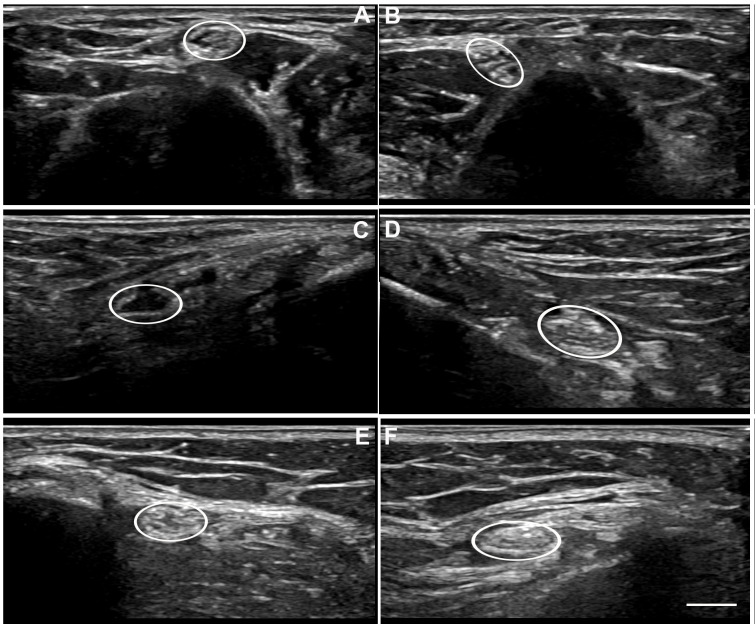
Representative ultrasonographic images of the nerves. Transverse ultrasonographic images of a normal ulnar nerve (A), a thickened ulnar nerve (B), a normal tibial nerve (C), a thickened tibial nerve (D), a normal common fibular nerve (E), and a thickened common fibular nerve (F), acquired with an ultrasound device Logiq P9. Scale bar: 0.5 mm.
